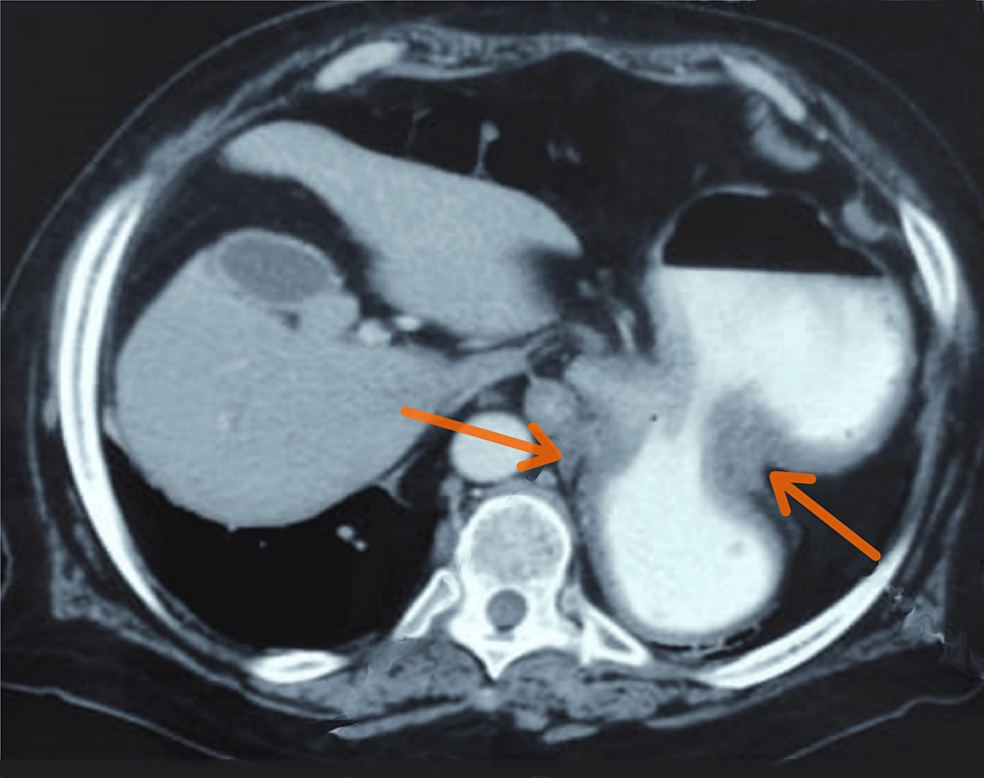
Axial section of the abdominal CT showing a distended stomach with torsion of the gastric body (two arrows) and upper air-fluid level.
